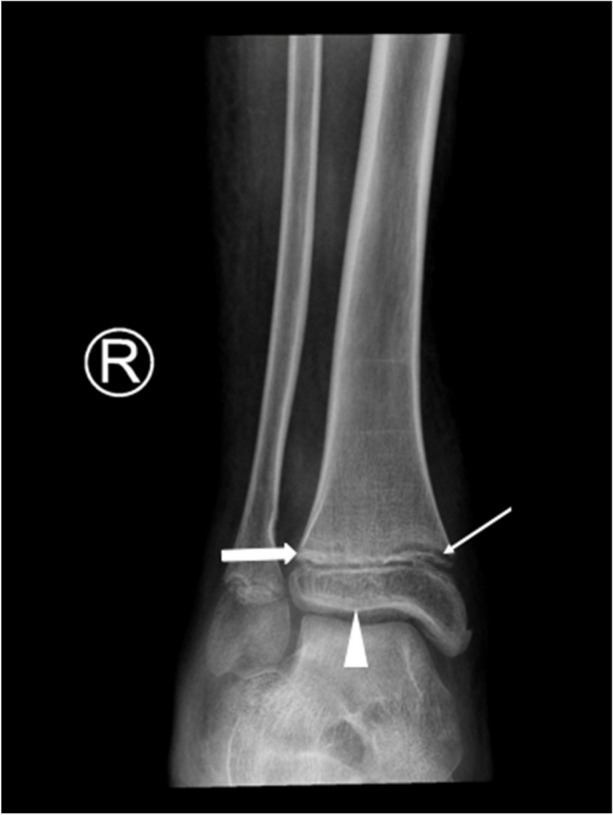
X-ray: X-ray of the right ankle shows Trummerfeld zone, white line of Frankel and Wimberger’s sign
